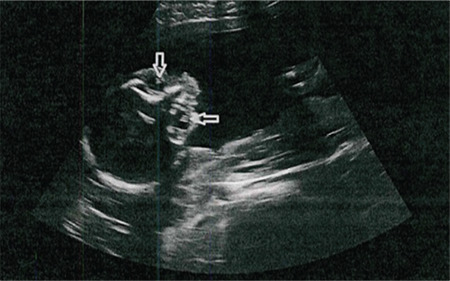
Ultrasound scan image of the fetus in utero at 21 weeks of gestation. Congenital cataracts appear as hyperechoic discs within the orbits (arrows)
